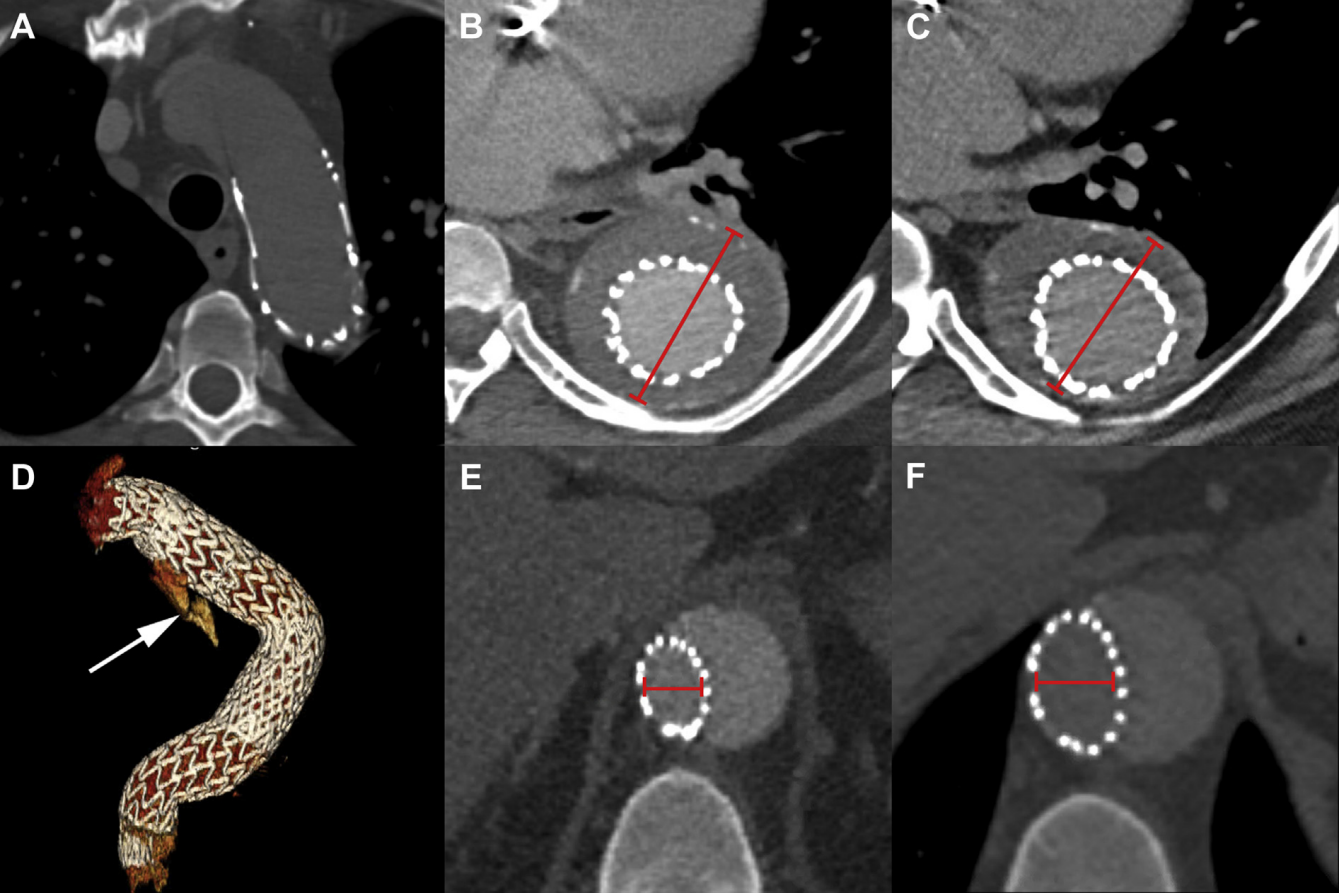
Follow-up computed tomography angiograms. A, Proximal seal zone at 6 months. B, Maximum diameter at 1 week measures 6.2 cm. C, Maximum diameter at 6 months measures 5.6 cm. D, Three-dimensional reconstruction of repair; *arrow* notes type II endoleak. E, Distal seal zone at 1 week measures 15 mm. F, Distal seal zone at 6 months measures 17 mm.
